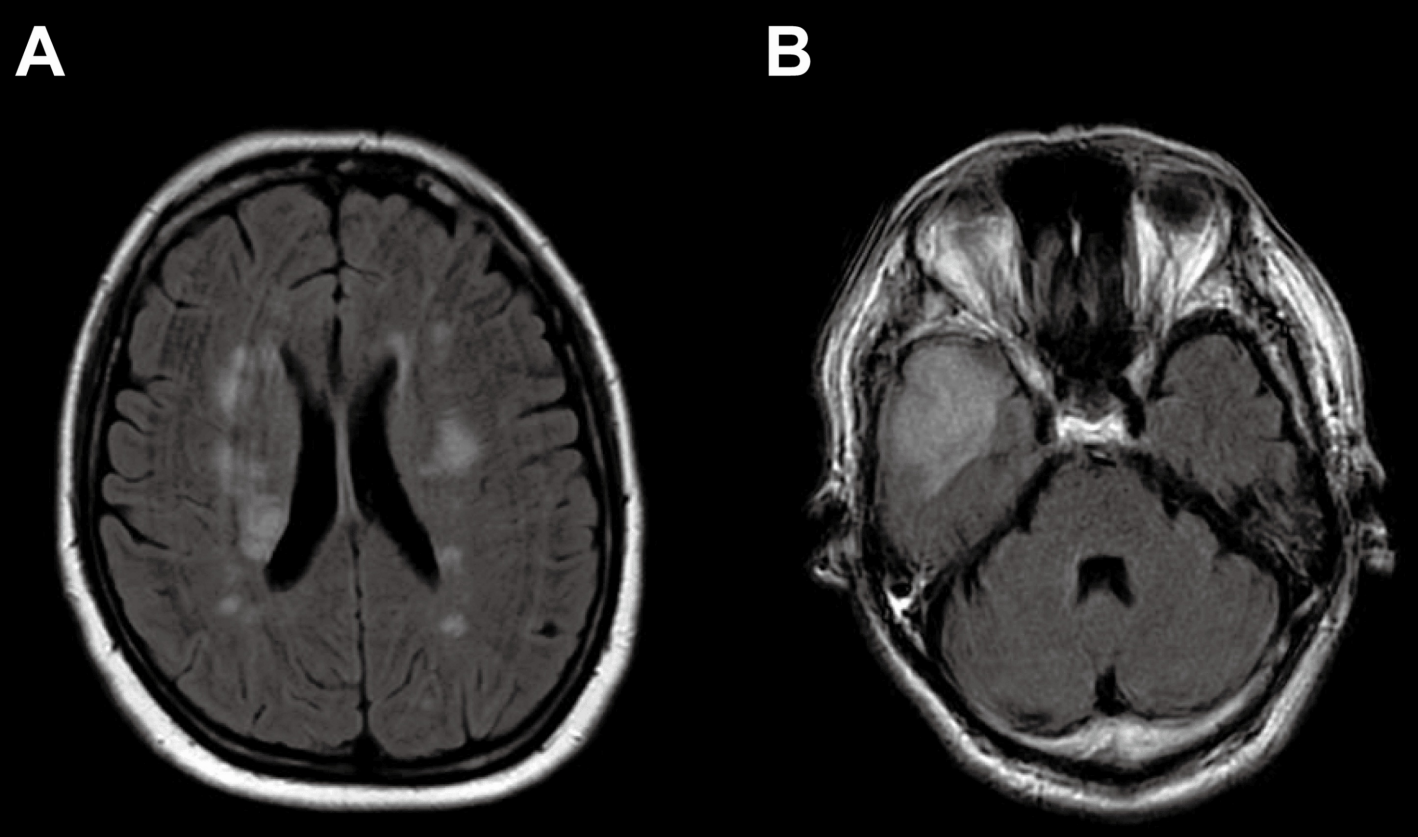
Postoperative MRI of the head in patients with newly developed stroke after TAR using DHCA. (A) Well-margined lacunar infarctions in multiple vascular territories, which were more prevalent in embolic stroke. (B) Cerebral malperfusion, exclusively defined as infarction in the territory of the cerebral artery associated with AAD in the presence of high-grade stenosis or lack of opacification through the arch vessels. MRI, magnetic resonance imaging; TAR, total arch replacement; DHCA, deep hypothermic circulatory arrest; AAD, acute aortic dissection.
